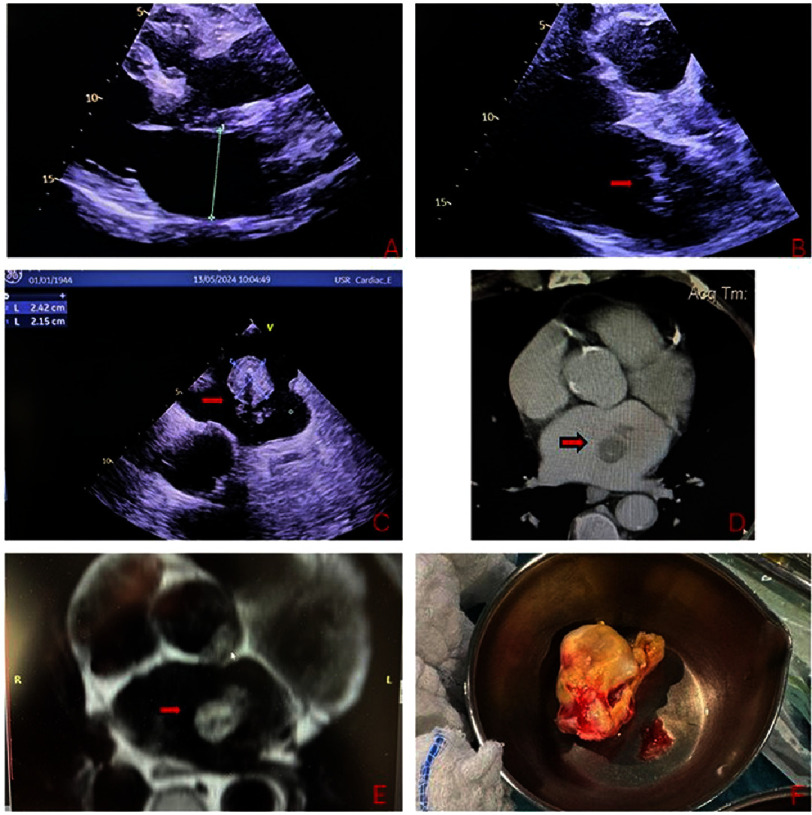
A: Transthoracic heart echo, long axis view depicting the absence of cardiac mass during the first evaluation of the patient (6 months ago). 1B: Transthoracic heart echo, long axis view depicting the presence of the cardiac mass (red arrow) during the present echo study of the patient. 1C: Transesophageal heart ultrasound depicting the presence of the intracardiac mass (red arrow), dimensions 2,15×2,4 cm. 1D: Cardiac computed tomography angiography depicting the cardiac mass within the left atrium (red arrow). 1E: Cardiac Magnetic resonance depicting the presence of the cardiac mass within the left atrium with low signal on both T1, T2 sequences (red arrow). 1F: Cardiac mass appearance after its surgical removal.
